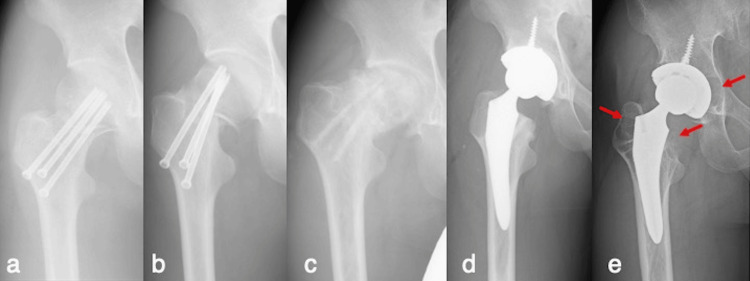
Anteroposterior radiographs of the right hip. (a) After internal fixation for a right femoral neck fracture 10 years ago; (b) femoral head displacement at two weeks; (c) necrotic femoral head after one year. The implant was removed; (d) post right total hip arthroplasty performed after two years; (e) extensive progressive osteolysis after seven years (arrows).
